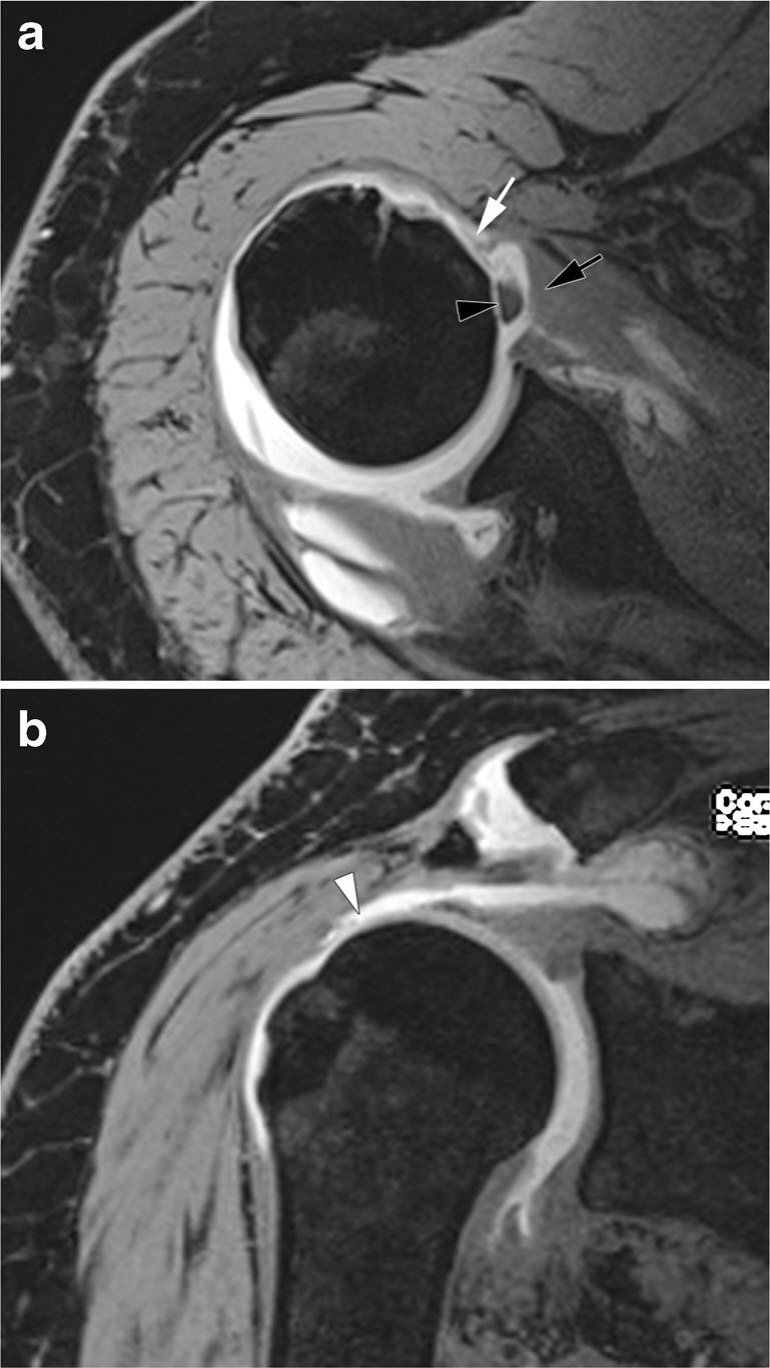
Axial (a) and sagittal oblique reformats (b) from a 3D FS T1-weighted MRA. Massive rotator cuff tear as shown by complete full thickness tears of the SSC (white arrow) (a) and the SSP (white arrow head) tendons (b). LHBT is medially dislocated (black arrow head) (a), and there is a proximal retraction of SSC tendon (black arrow)
